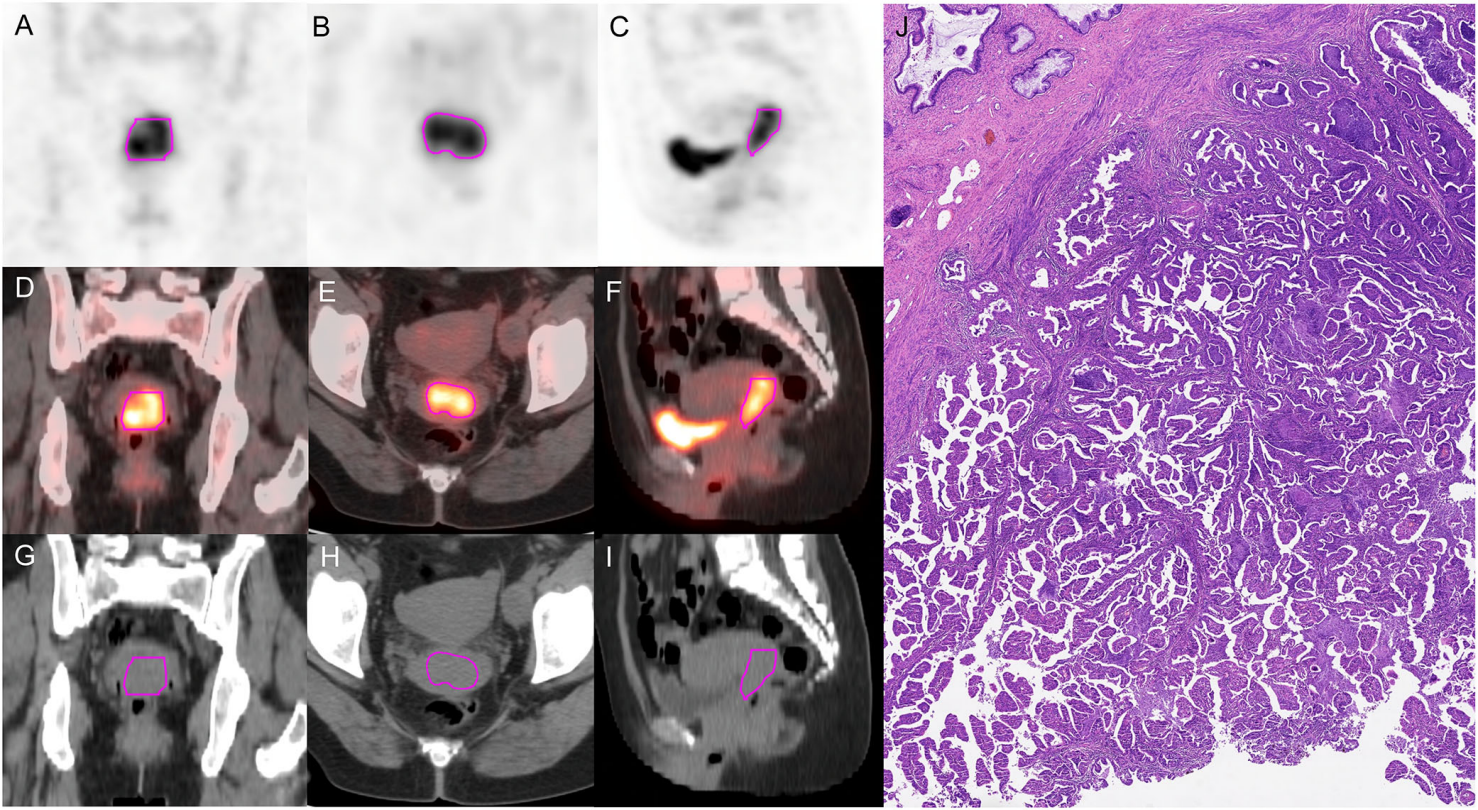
A 41-year-old woman diagnosed with AC. (A–C) The ROI, labeled in red, was segmented on the coronal, axial, sagittal PET images. (D–F) The ROI, labeled in red, was segmented on the coronal, axial, sagittal fusion images. (G–I) The ROI, labeled in red, was segmented on the coronal, axial, sagittal CT images. (J) Pathological examination confirmed AC.
